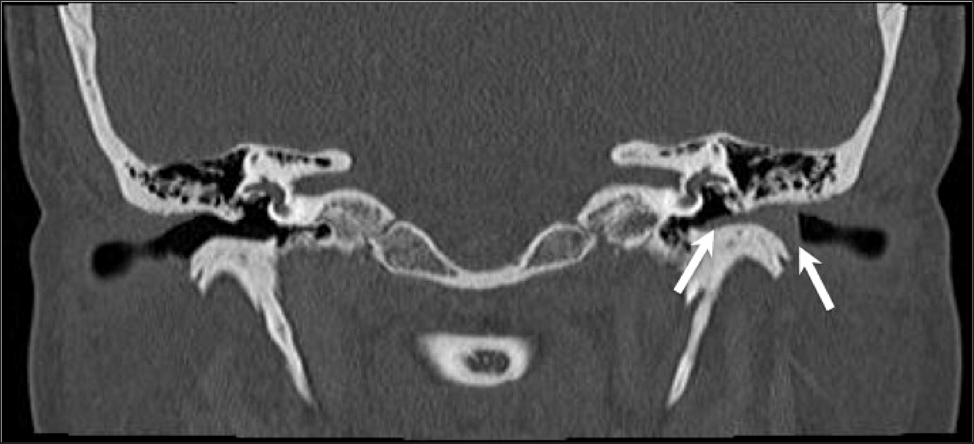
Coronal HRCT image shows totally obstructed left EAC by soft tissue density mass (arrows) without bony EAC erosion or destruction. The middle and internal ear structures are normal.
